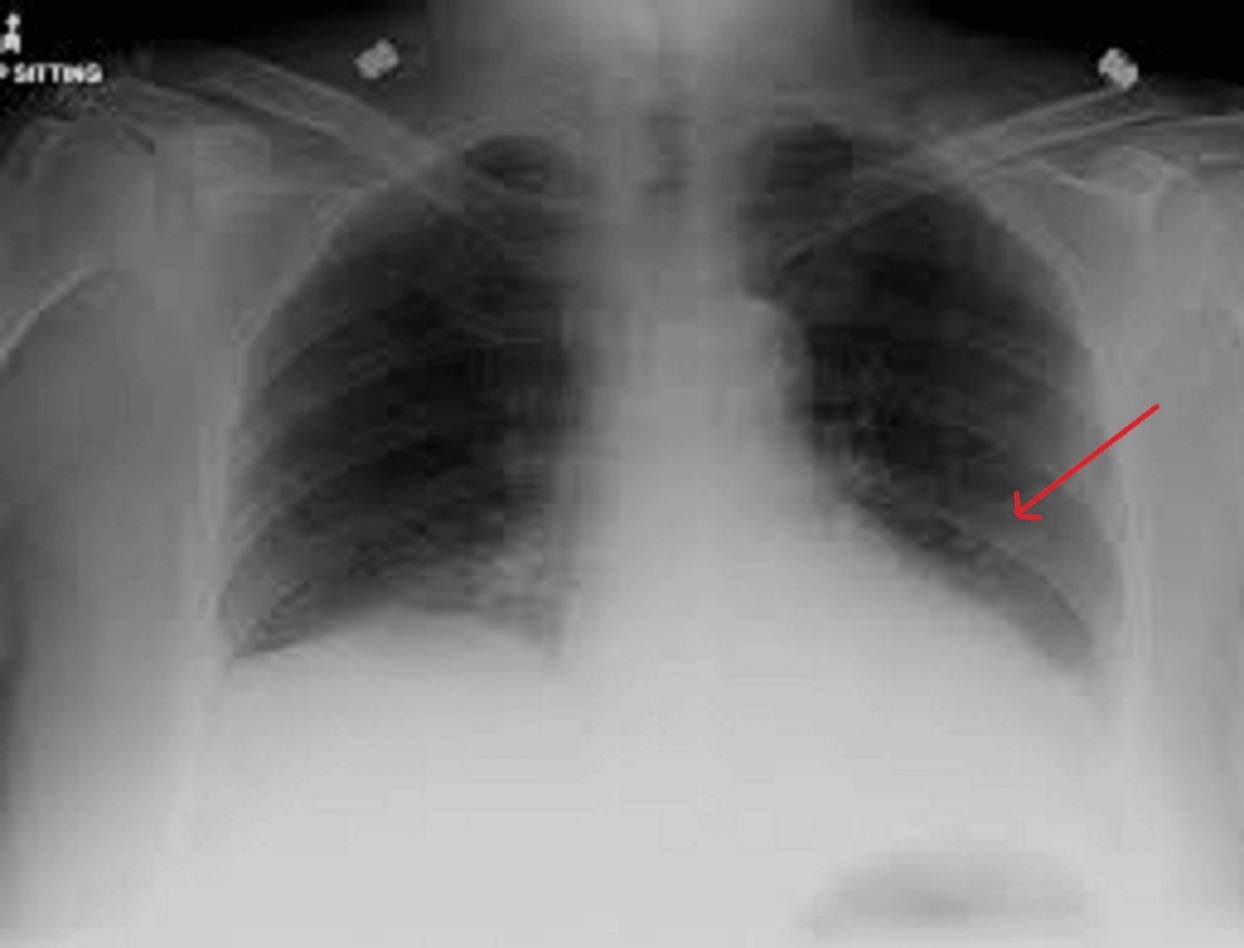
Chest X-ray (posteroanterior (PA) view) showing left lower lobe consolidation. The red arrow indicates the area of consolidation. Source: Radiology department, Port of Spain General Hospital
